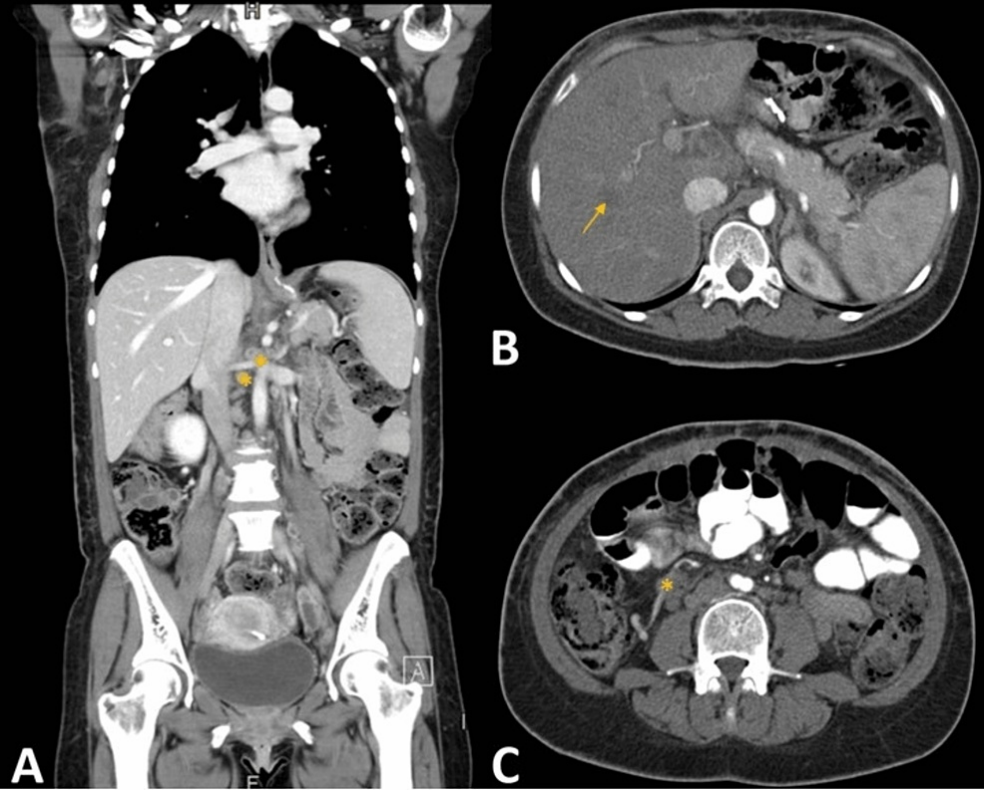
CT showing mediastinum, celiac, mesenteric, and latero-aortic adenomegalies, with necrotic center (*), and hepatosplenomegaly with hepatic nodular areas (arrow) (A) Coronal plane. (B) Transverse plane at the liver level. (C) Transverse plane at a lower level CT: computed tomography
